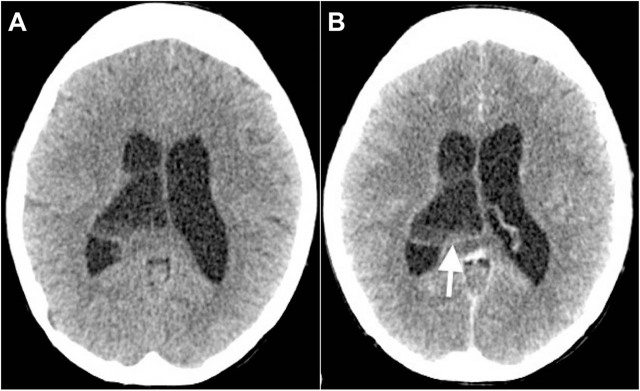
Axial images of (A) plain and (B) contrast computed tomography of the brain show a cystic lesion within the right lateral ventricles with sediments and septations within. There is also a minimal rim enhancement of the wall (arrow) and dilatation of both lateral ventricles consistent with hydrocephalus.
